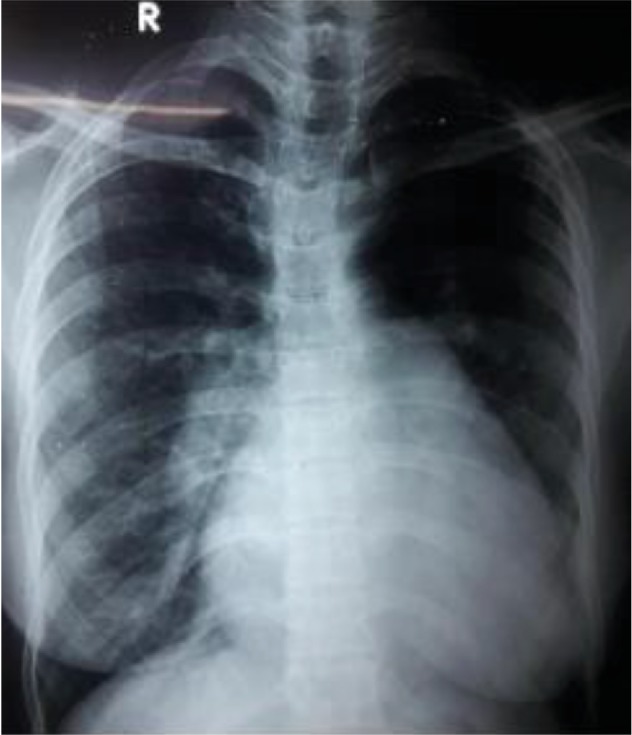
Chest X-ray P/A view showing bulged pulmonary conus, cardiomegaly, and prominent right pulmonary artery.
